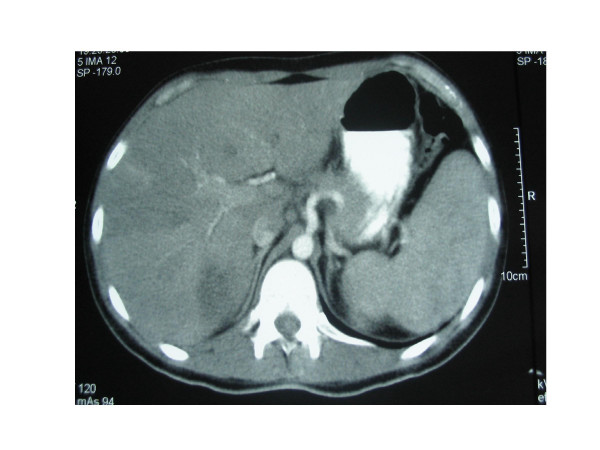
Abdominopelvic contrast-enhanced computed tomography. Multiple liver abscesses and mesenteric partial thrombosis.
